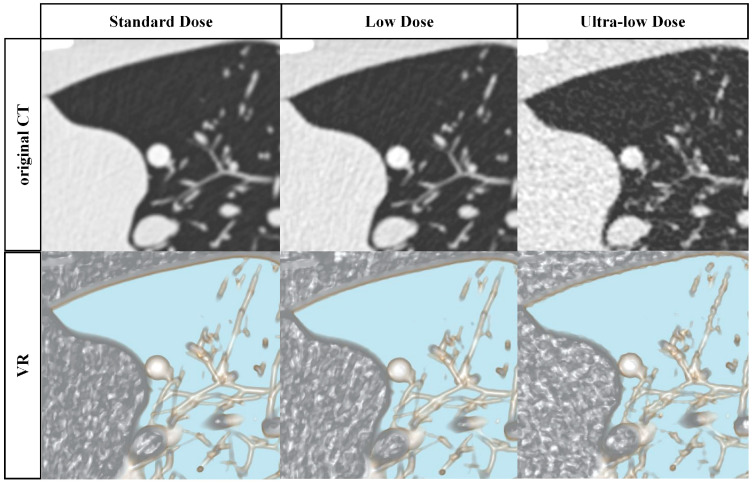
Representative chest CT images and corresponding volume rendering (VR) images from standard-dose computed tomography (SDCT), low-dose computed tomography (LDCT), and ultra-low-dose computed tomography (ULDCT), reconstructed using the Br40 kernel and ADMIRE−3 algorithm. The images reveal a solid nodule in the left upper lobe with a CT attenuation of 100 HU. The solid nodule appears clearer and more detailed in the axial CT images under SDCT and LDCT scanning compared with ULDCT. However, the nodule remains distinctly visible in the VR images, even under ULDCT scanning. Ultimately, the nodule was missed in both the axial CT images and the CAD2 analysis.
